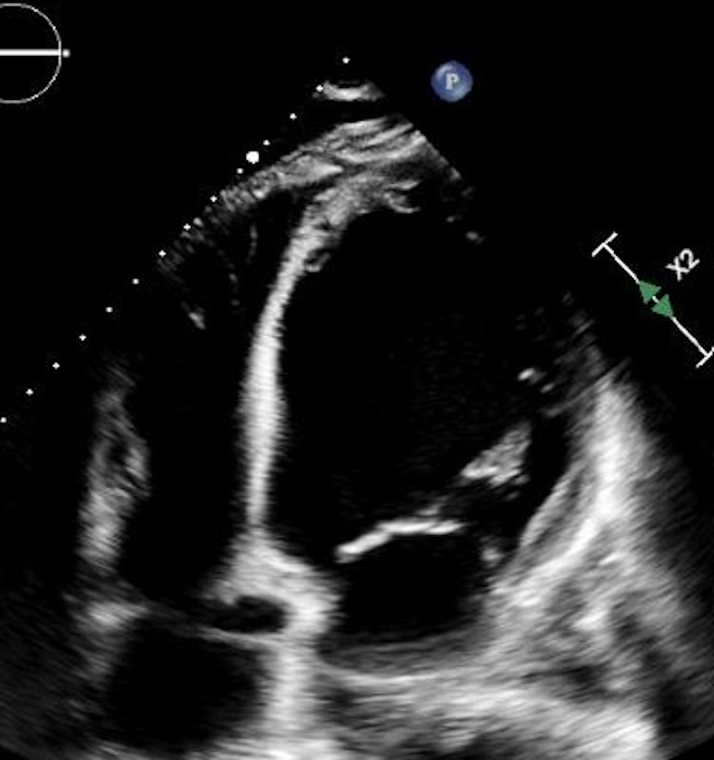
Transthoracic Echocardiogram Demonstrating Severe Biventricular Systolic Dysfunction With Global Hypokinesis and an Estimated Left Ventricular Ejection Fraction of 15% to 20%
